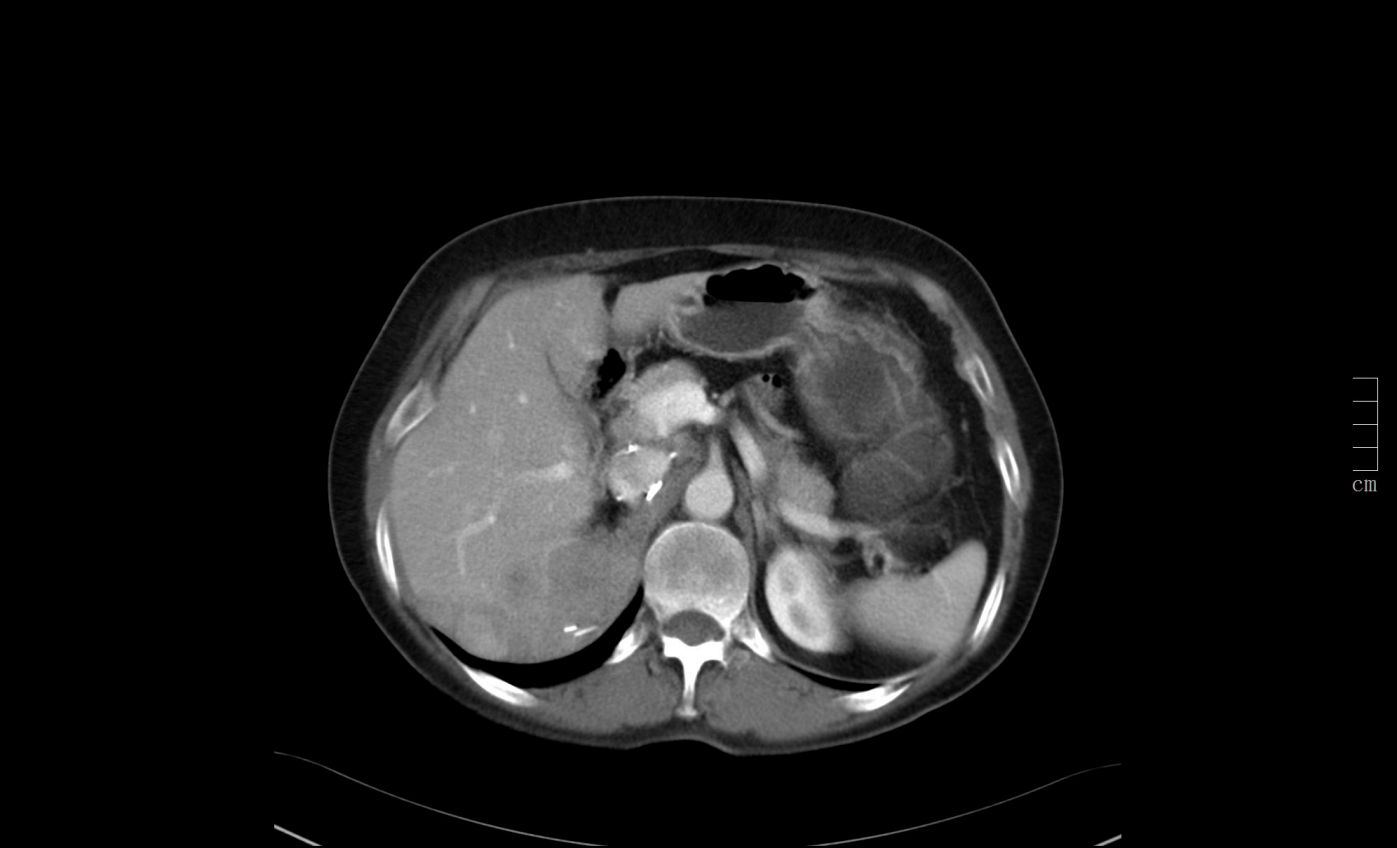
Metastatic spread of pheochromocytoma in abdominal CT
